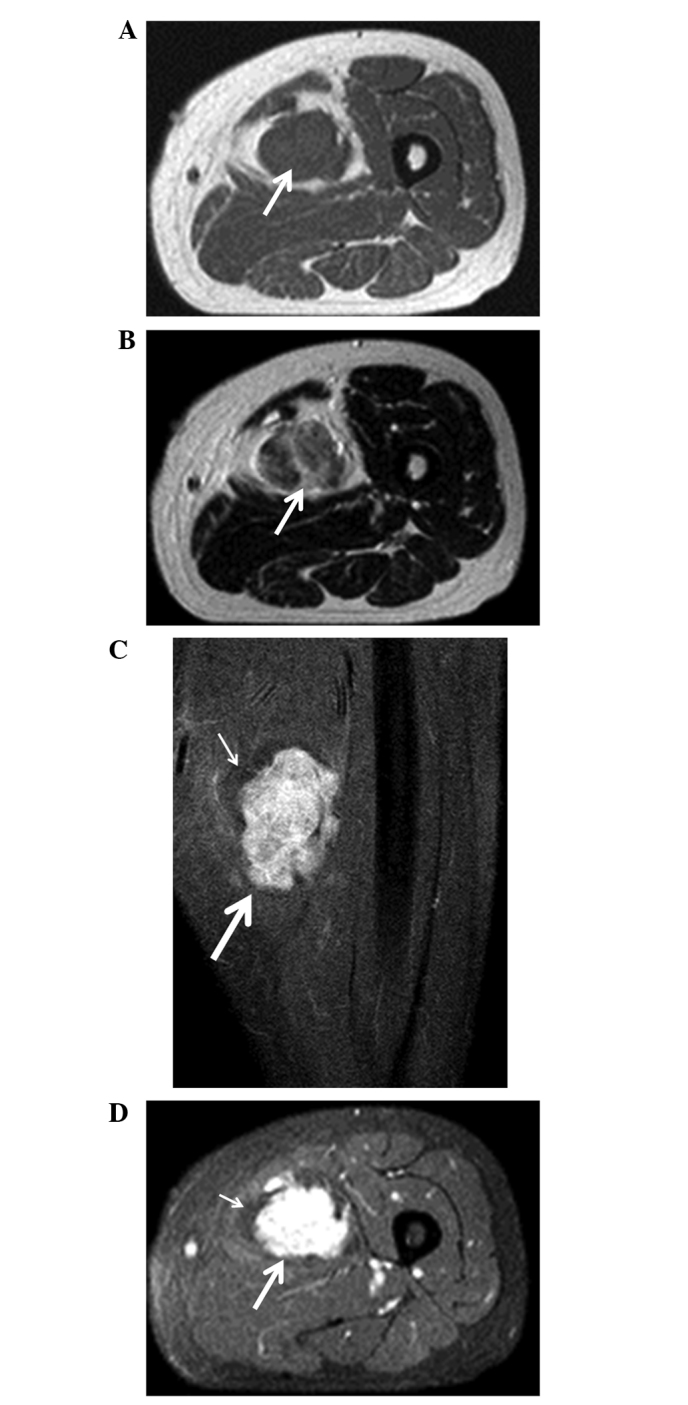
Magentic resonance imaging (MRI) findings on (A) T1- and (B) T2-weighted axial imaging revealing an intramuscular, low signal intensity mass located in the adductor longus (arrow). (C) Fat-suppressed gadolinium-enhanced frontal and (D) axial imagings revealing a multinodular, homogeneous, high signal intensity mass (large arrow). The surroundings of the mass are not enhanced (small arrow).
